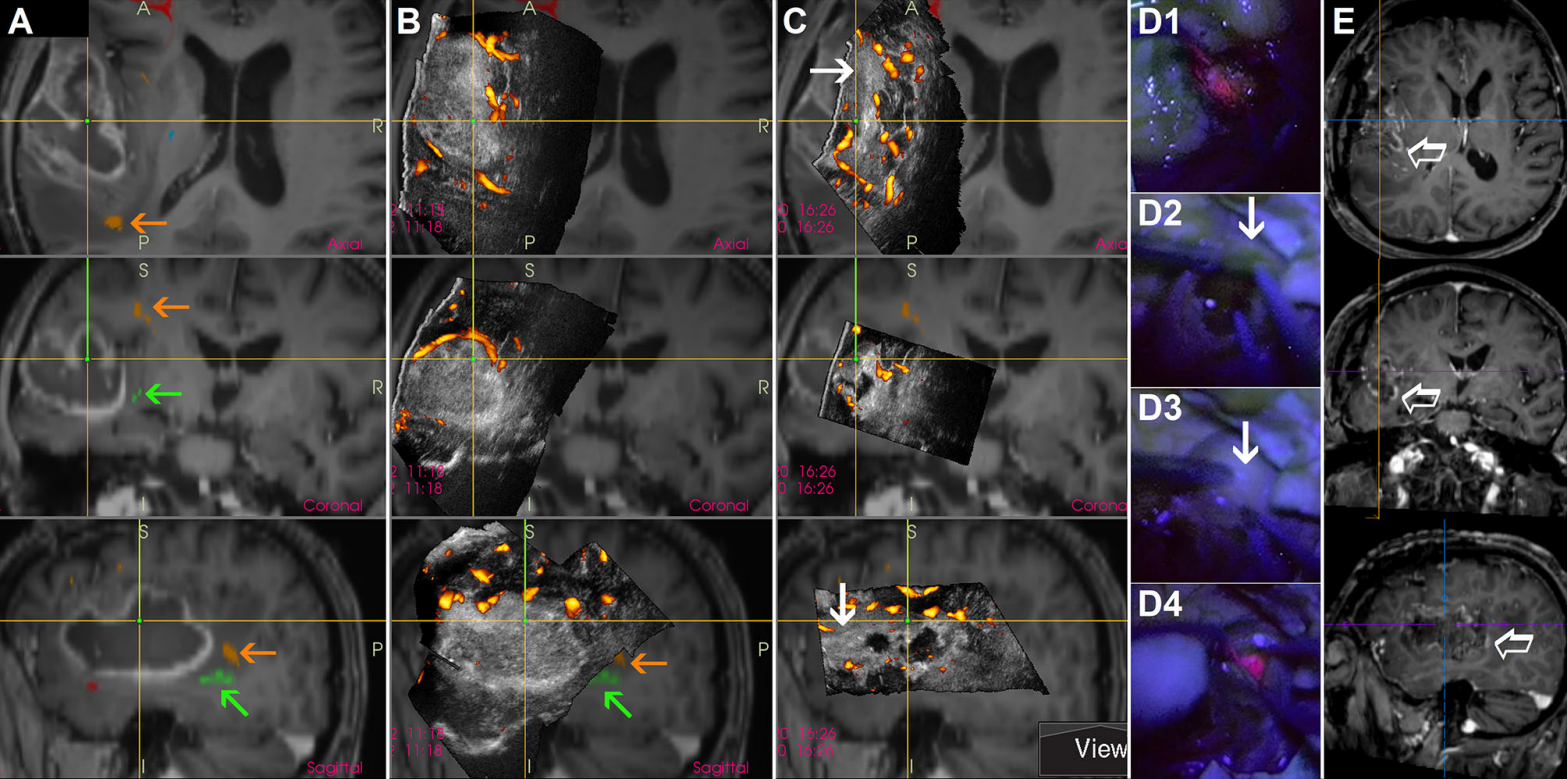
Awake resection of a left-sided temporal glioblastoma guided by direct electrical stimulation, navigated intraoperative 3D IOUS and 5-aminolevulinic acid (A) preoperative navigation 3D contrast-enhanced T1-weighted MRI sequence with implemented tractography. Orange arrows = arcuate fascicle. Green arrows = inferior fronto-occipital fascicle (B) Pre-resectional 3D IOUS image fused with navigation MRI. Orange arrows = arcuate fascicle. Green arrows = inferior fronto-occipital fascicle (C) 3D IOUS image acquired during the resection. Despite the absence of red fluorescence in the anterior part of the resection cavity at the time of IOUS scanning, ultrasound image showed a large nodular tumor residuum in this area (arrows) (D1) Distinct red fluorescence observed shortly after the resection beginning (D2, D3) Absence of red fluorescence in the anterior part of the resection cavity. Arrow—presumably high grade tumor part identified using actual 3D IOUS scans (D4) Distinct red fluorescence after cortical resection (E) Postoperative MRI performed 72 hours after the surgery. Empty arrow—contrast-enhancing residual tumor intentionally left in place, electrical stimulation of inferior fronto-occipital fascicle in this area elicited semantic paraphasias. 3D, three-dimensional; MRI, magnetic resonance imaging; IOUS, intraoperative ultrasound.
